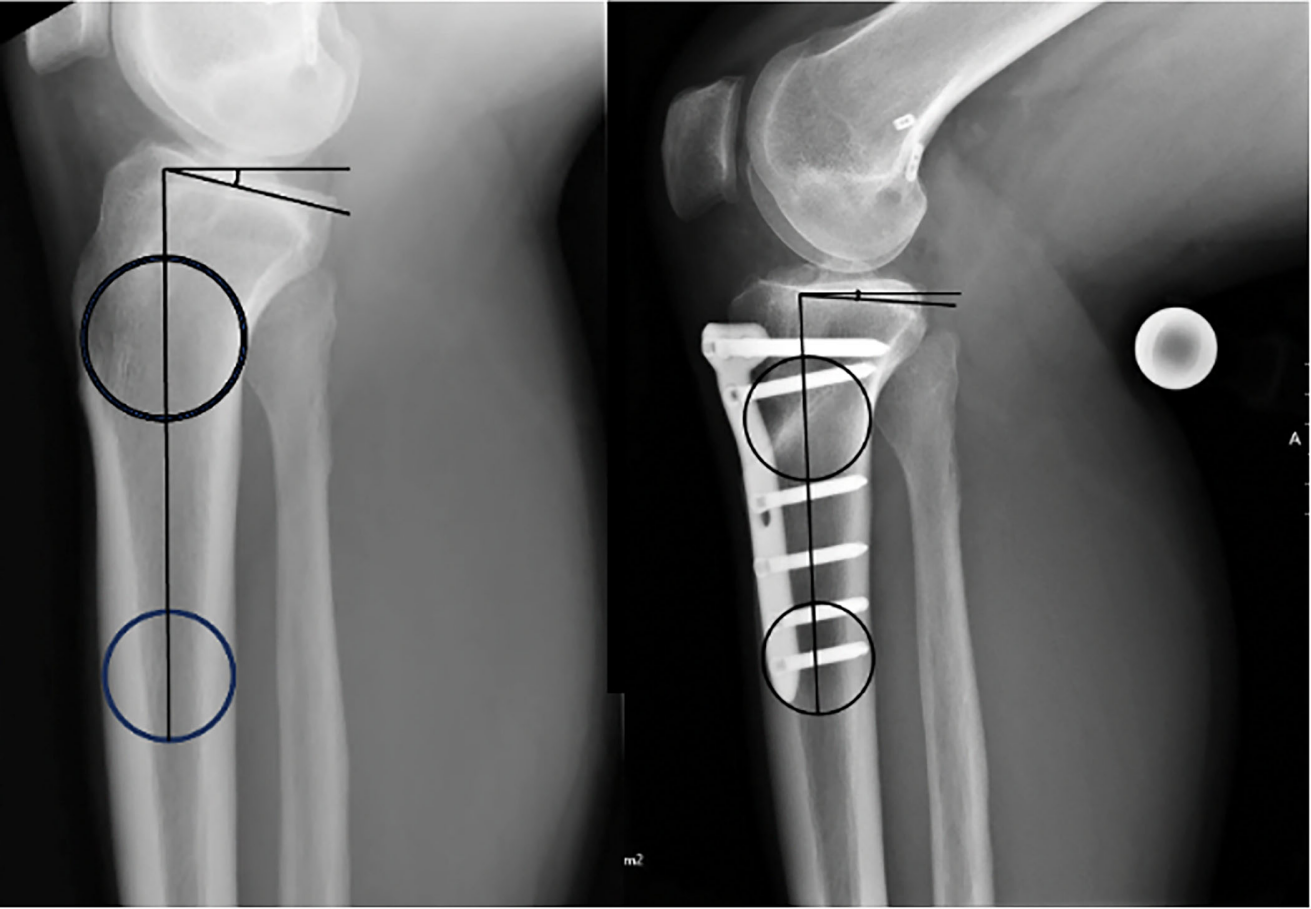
Preoperative (24.5° PTS) and postoperative (7.0° PTS) radiographs before and after slope reducing high tibial osteotomy. PTS, posterior tibial slope.
